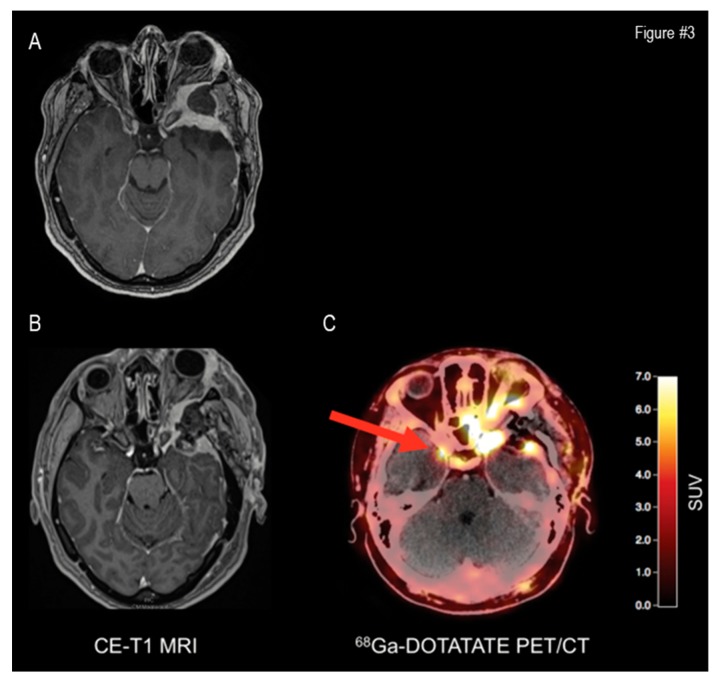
Additive value of SSTR-PET to MRI in restaging. A 42-year-old patient with a history of a left sphenoid skull base meningioma. Preoperative MRI shows tumour recurrence (Panel A). Postoperative MRI (Panel B) shows an incomplete resection of the tumour, necessitating adjuvant radiotherapy. 68Ga-DOTATATE PET/CT reveals an additional meningioma located at the tip of the right sphenoid wing (Panel C). *Reprinted with permission from Galldiks et al.* [78].
